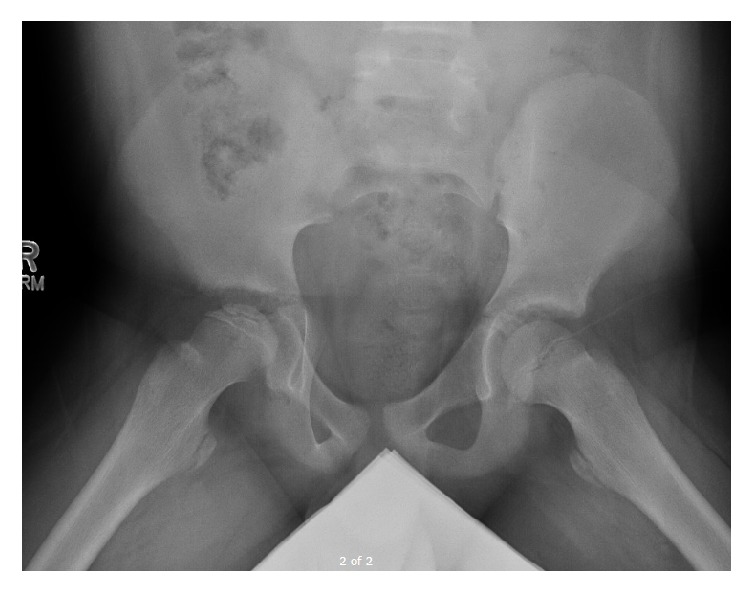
Frog leg lateral X-ray of bilateral hips again showing avascular changes with characteristic “crescent sign” of osteonecrosis to right femoral head.
